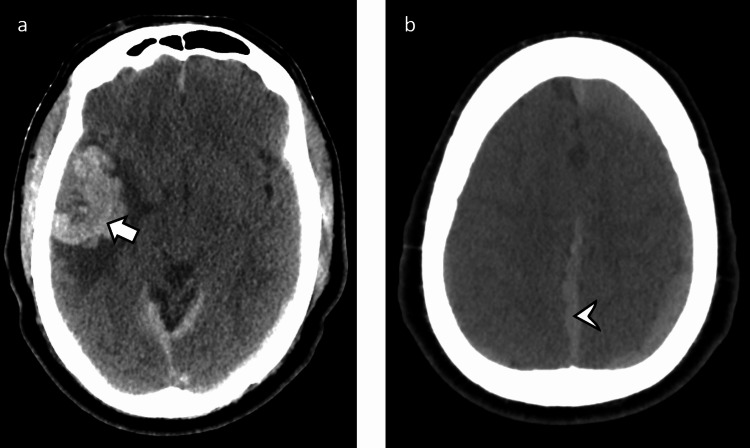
CT head without contrast a: Large right temporal intraparenchymal hemorrhage (arrow) with surrounding edema as well as partially visualized subdural hemorrhages along the anterior falx and bilateral tentorium. b: Subdural hemorrhage along the falx (arrowhead) and bilateral cerebral convexities CT: computed tomography
